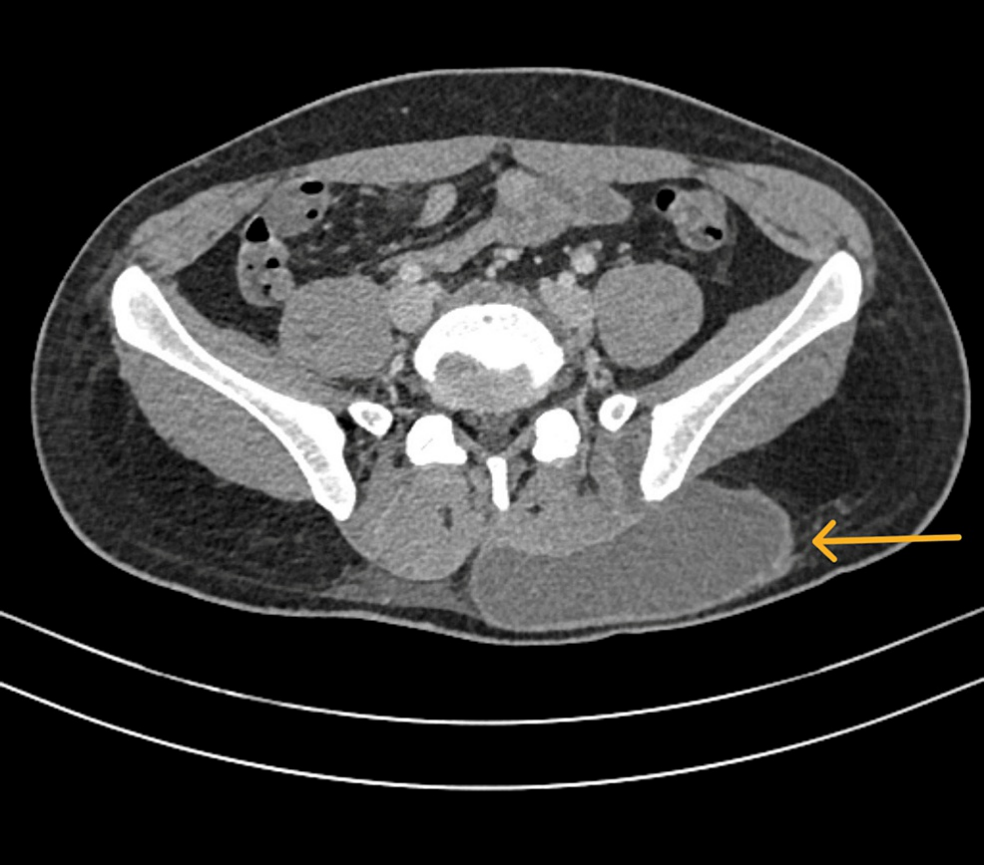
Axial cut CT of the abdomen showing left gluteal/paraspinal collection measures approximately 12 cm × 6.5 cm, with anterior and posterior vertebral extension.
